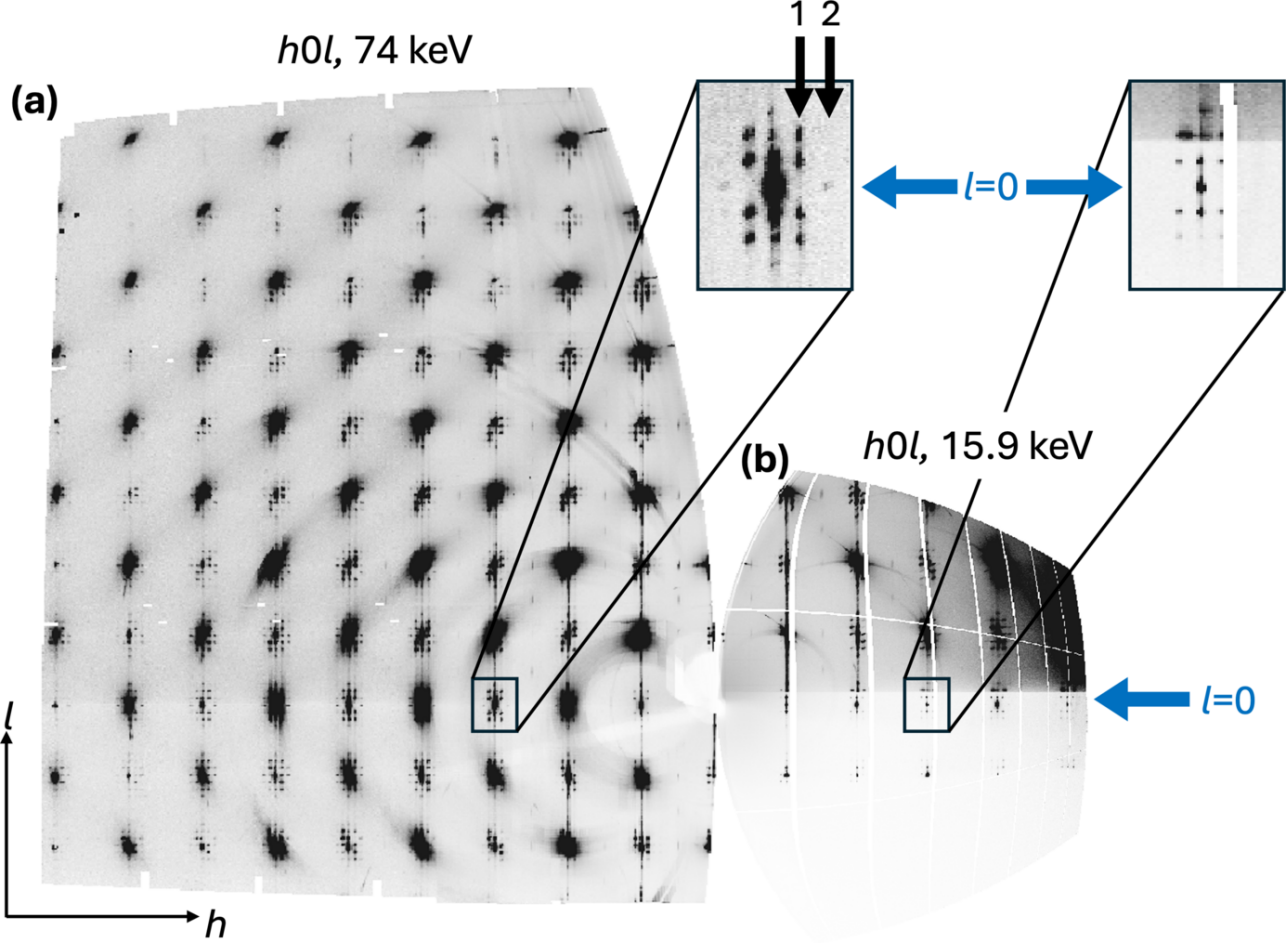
(*a*) Reconstruction of *h*0*l* layers collected at an SDD of 493.7 mm, an incident angle of 0.03° and an X-ray energy of 74 keV, with detector gaps filled (HE-GID). (*b*) Reconstruction of *h*0*l* layers collected at an SDD of 143 mm, an incident angle of 2° and an X-ray energy of 15.9 keV (ME-GID). Here, the detector gaps are not filled. The magnified insets show the corresponding reflection environments from the two experiments. In these insets, the HE-GID recorded
